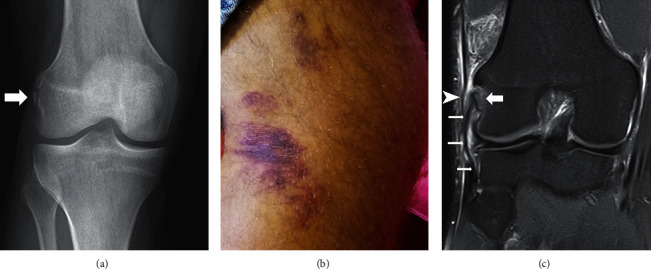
Patient case 1. (a) AP right knee X-ray at day 1 showing the avulsion fracture of the femoral insertion of the LCL (white arrow). (b) Clinical appearance at 2 weeks showing bruises over lateral aspect of the right knee. (c) Proton density coronal MRI of the right knee at day 3 showing a wavy though intact LCL (small arrows) attached to the avulsed bony fragment of the femoral insertion (large arrow). The iliotibial band (arrowhead) lies immediately superficial to the proximal LCL.
